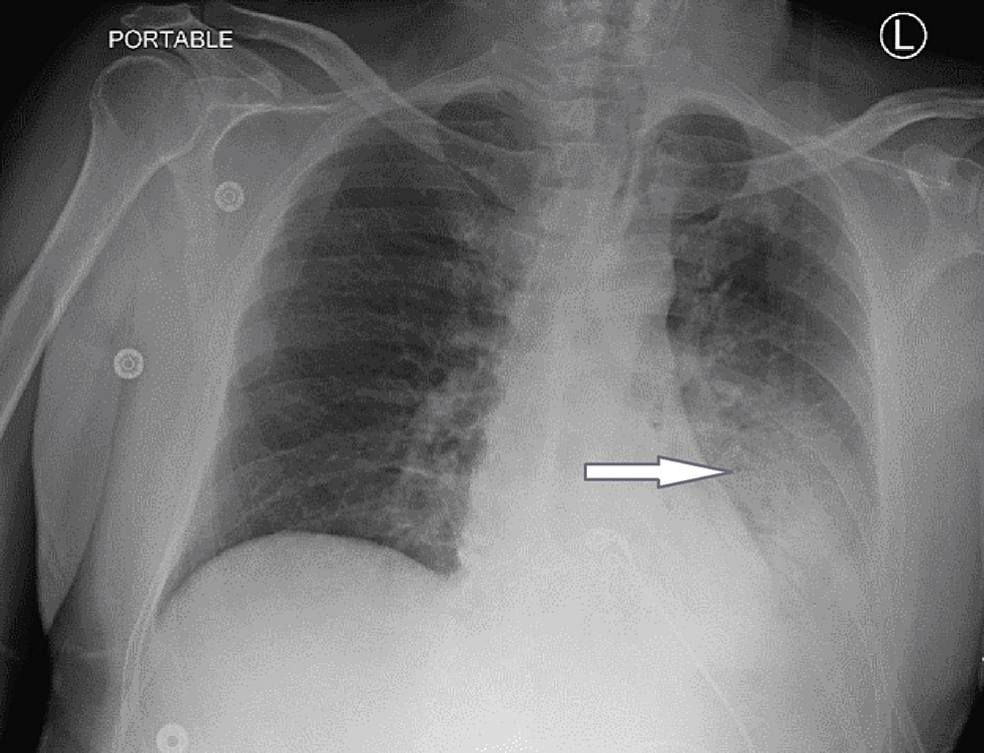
Chest X-ray showing pulmonary infiltrates on the left The blue arrow indicates an area of ill-defined infiltrates in the lower lobe of the left lung.
